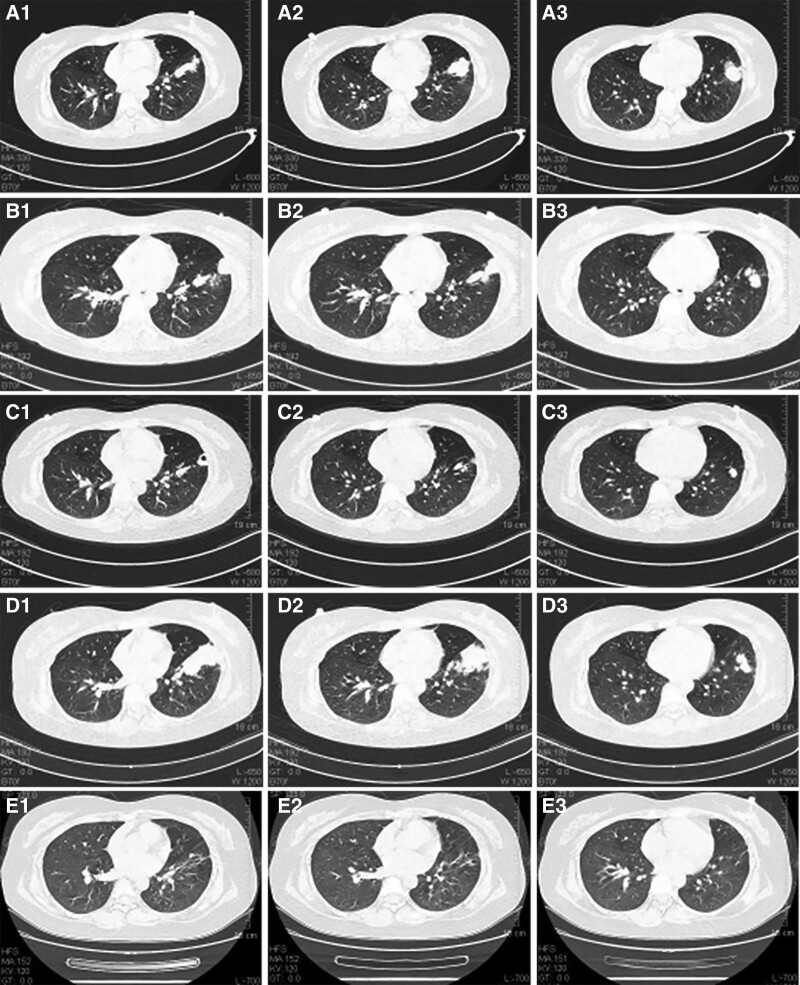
(A) Chest CT (2019-8-26) revealed left lower lobe pathological changes. (B) Chest CT (2019-10-4) showed progression of the lesion. (C) Chest CT (2020-1-2) showed that the exudation was obviously absorbed and cavity formed compared with the previous 1. (D) Chest CT (2020-5-19) showed progression of the lesion. (E) Chest CT (2020-10-27) showed that the focus of infection had been obviously absorbed. CT = computer tomography.
